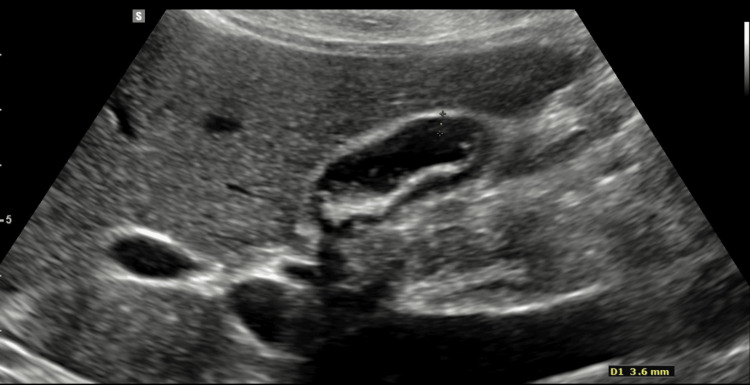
Patient's ultrasound image of thin-walled gallbladder containing stones
